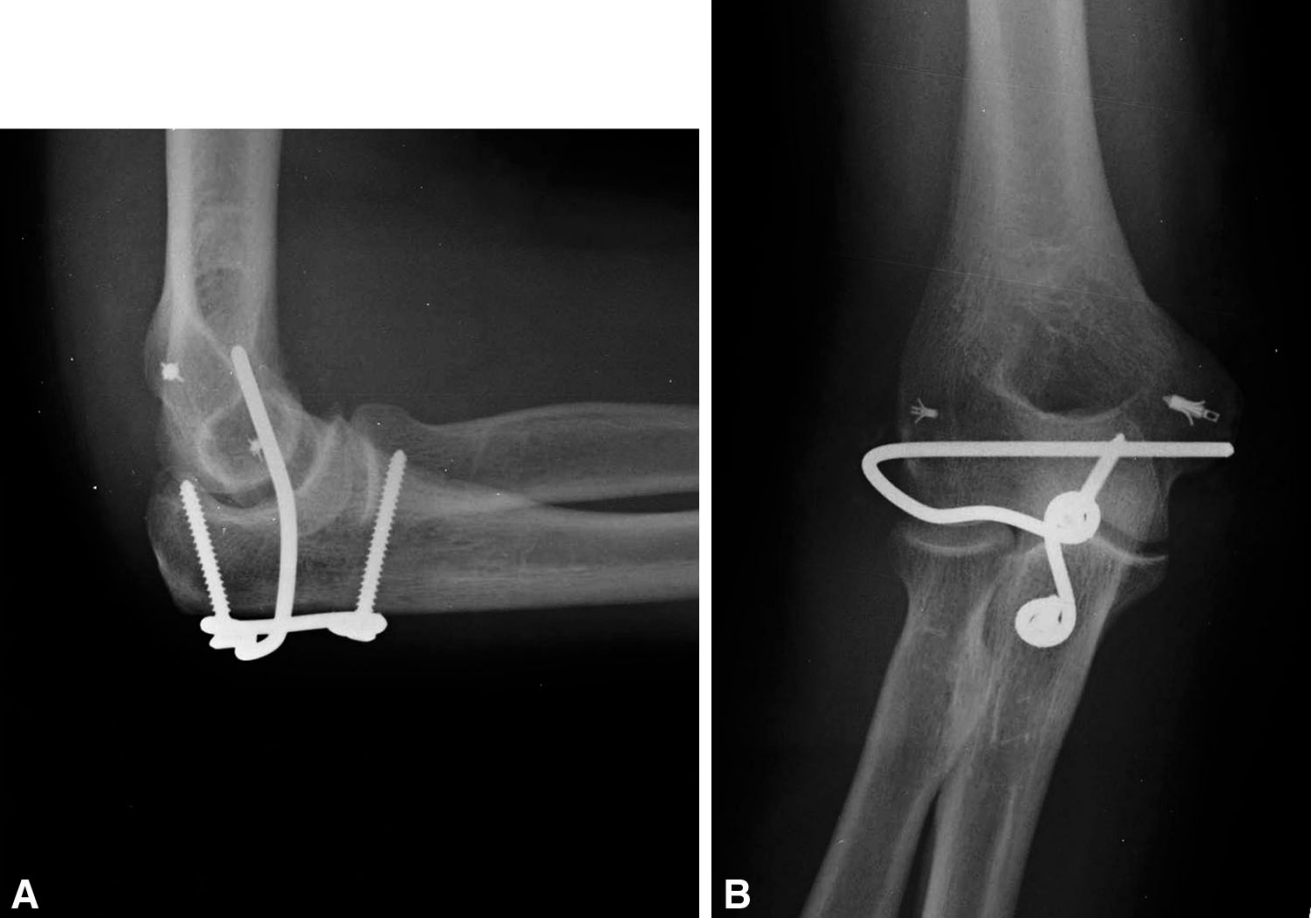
AP and lateral radiographs show the joint stabilizer. (A) The implant is attached to the ulna by means of two 3.5-mm compression screws. (B) The axis portion of the implant traverses the distal humerus in line with the axis of ulnohumeral rotation.
